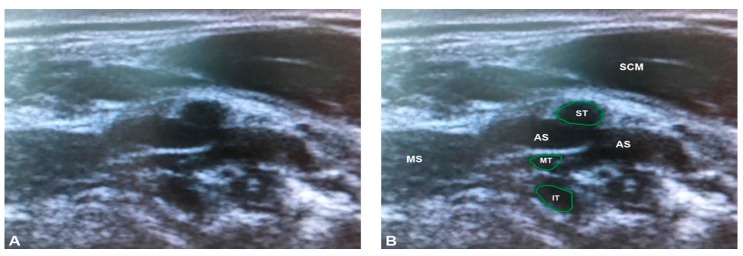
The anterior variant, identified using ultrasonography in the present study: (A) unlabeled; and (B) labeled. Note that the superior trunk courses superficial to the anterior scalene muscle in this condition. AS = anterior scalene; IT = inferior trunk; MS = middle scalene; MT = middle trunk; SCM = sternocleidomastoid; ST = superior trunk. The green outlines demarcate the trunks of the brachial plexus.
